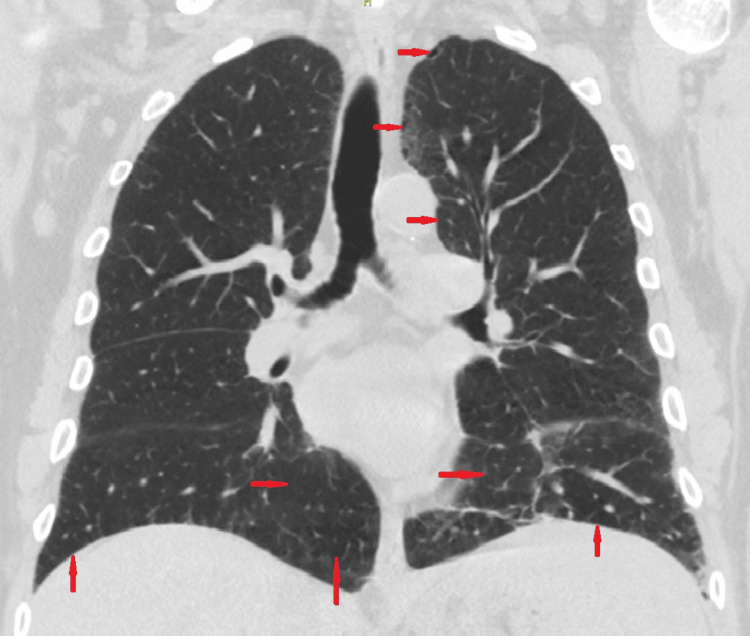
CT scan of the chest (coronal view) The red arrows indicate emphysematous changes seen in the lungs.
